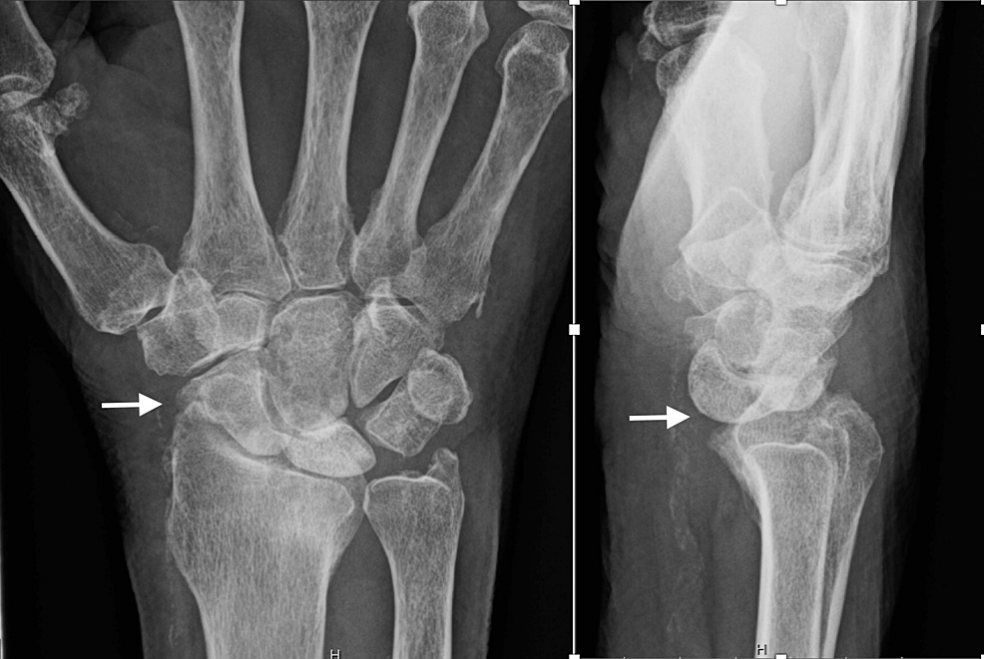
Preoperative radiographs of Case 2 A 90-year-old male with volar lunate subluxation with severe radiocarpal and midcarpal arthritis. The white arrows show the radiocarpal and midcarpal arthritis.
